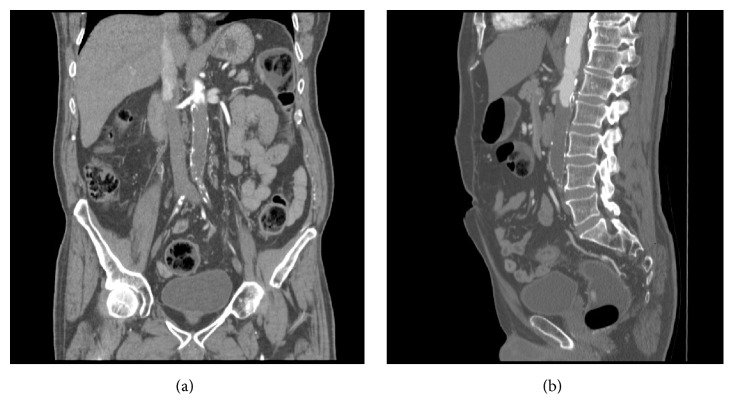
Coronal and sagittal CT angiography views, showing complete thrombosis of the aortoiliac axis.
